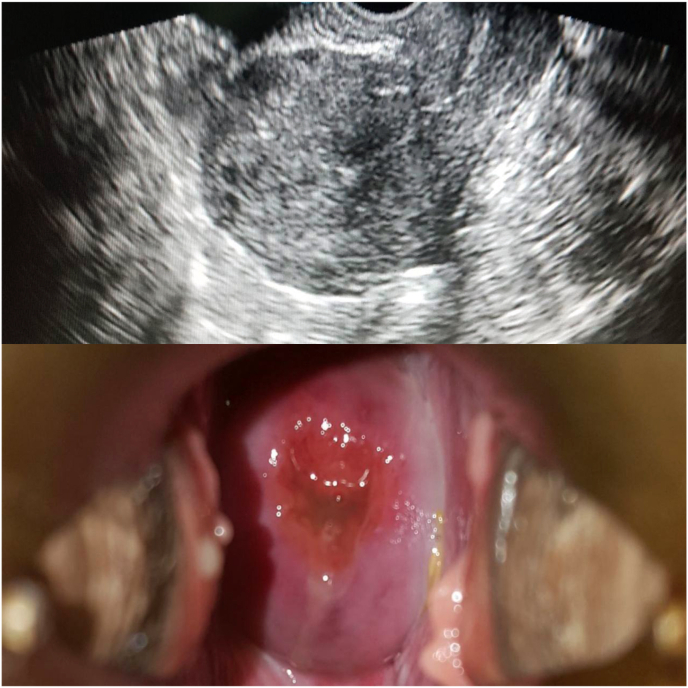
Per speculum examination and ultrasound showing normal anatomy of the cervix and vagine.
